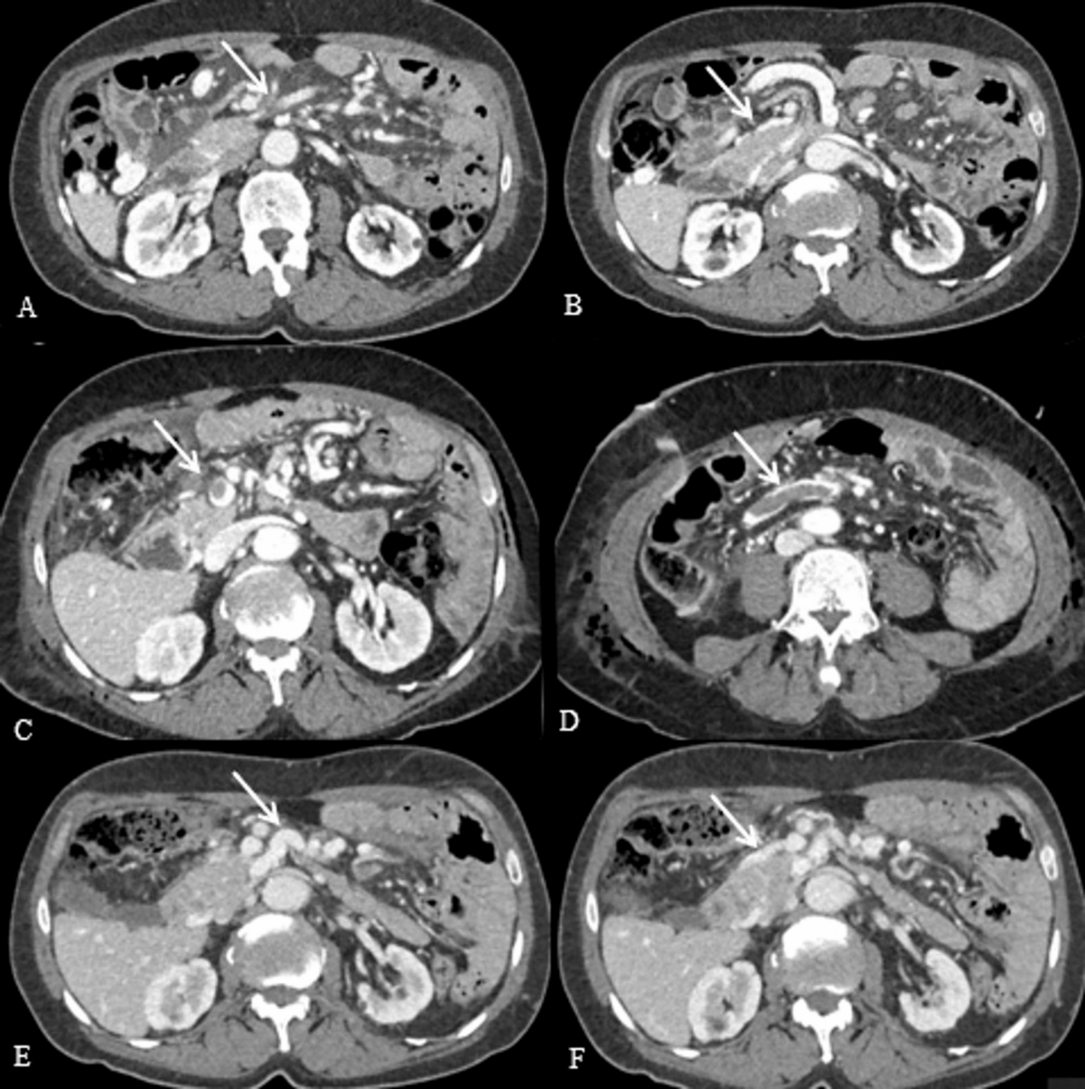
Contrast-enhanced computed tomography Coronal images revealing superior mesenteric vein filling defects, collaterals, and a partially enhanced thrombus before (A, B) and after (C, D) surgery. Three months postoperatively, after anticoagulation treatment, the superior mesenteric vein has been recanalized and no filling defects are visible (E, F).
